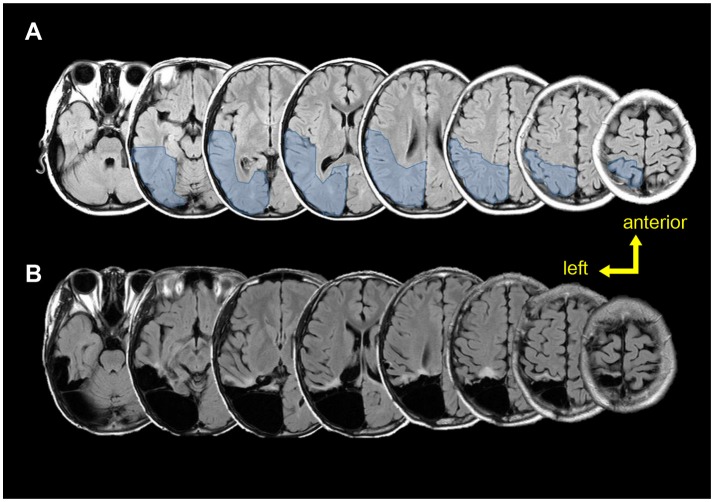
Pre-post-surgical T2 flair magnetic resonance images. (A) Pre-surgical images. Light blue region shows the planned resection range. (B) Post-surgical (12 months after surgery) images show the true resection range.
